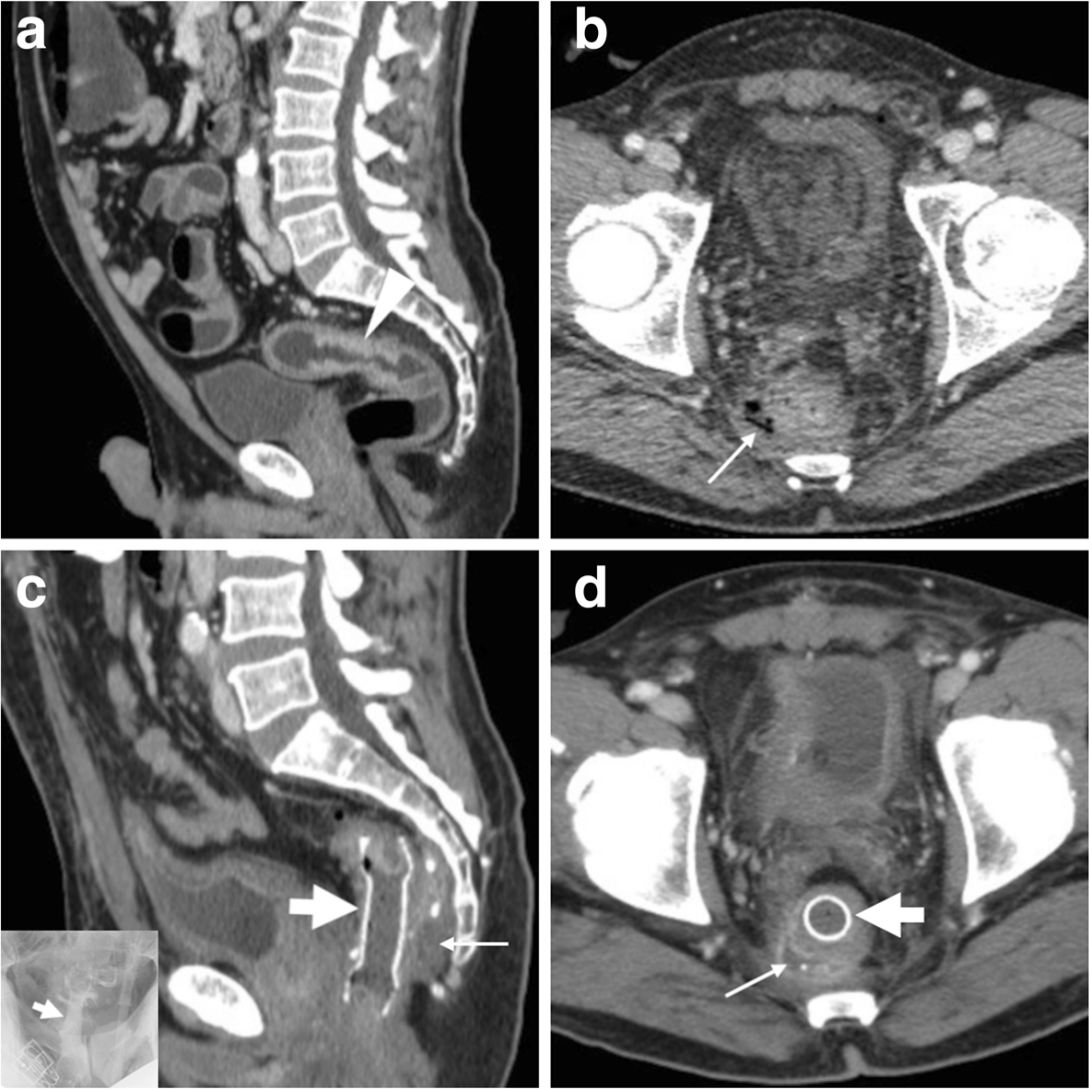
Successful stent management of postoperative AL. Preoperative water enema CT (a) showed stricturing CRC of the rectosigmoid junction (arrowhead). Days after anterior rectal resection with an ileostomy, CT (b) was requested because of fever and faecaloid material from drainage tube. Gas bubbles (thin arrow) and mild effusion abutting the site of coloanal anastomosis were consistent with the clinical and endoscopic diagnosis of AL, which was treated by placing a covered SEMS through the anastomosis (inset image in c). A week later, follow-up CT (c, d) showed SEMS (thick arrow) in place, minimal residual perianastomotic fluid collection (thin arrows) and the patient ultimately did well.
